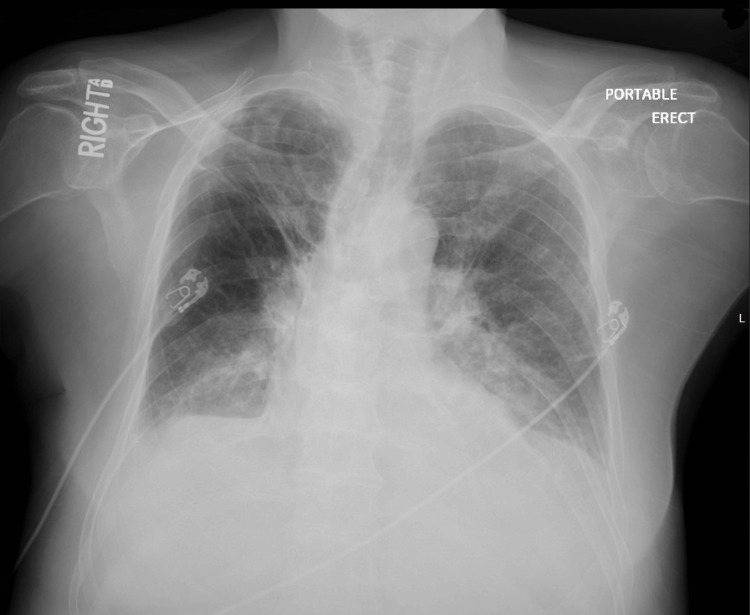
X-ray at the initial presentation to the emergency department
